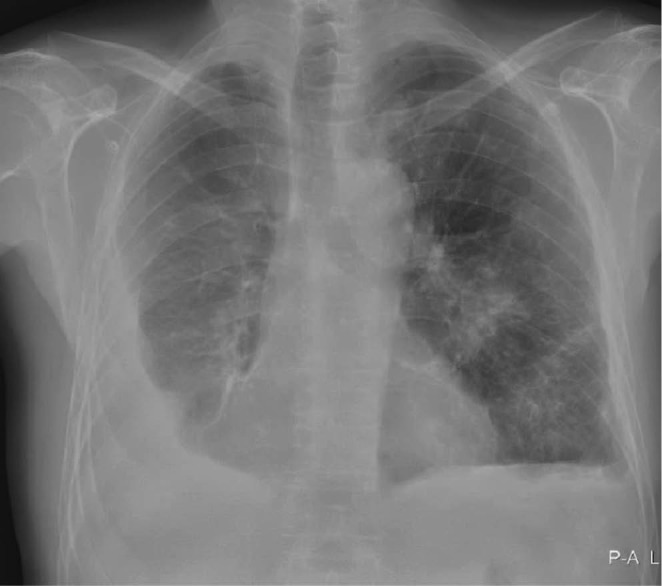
Chest X-Ray after patient presentation at the Emergency department.
